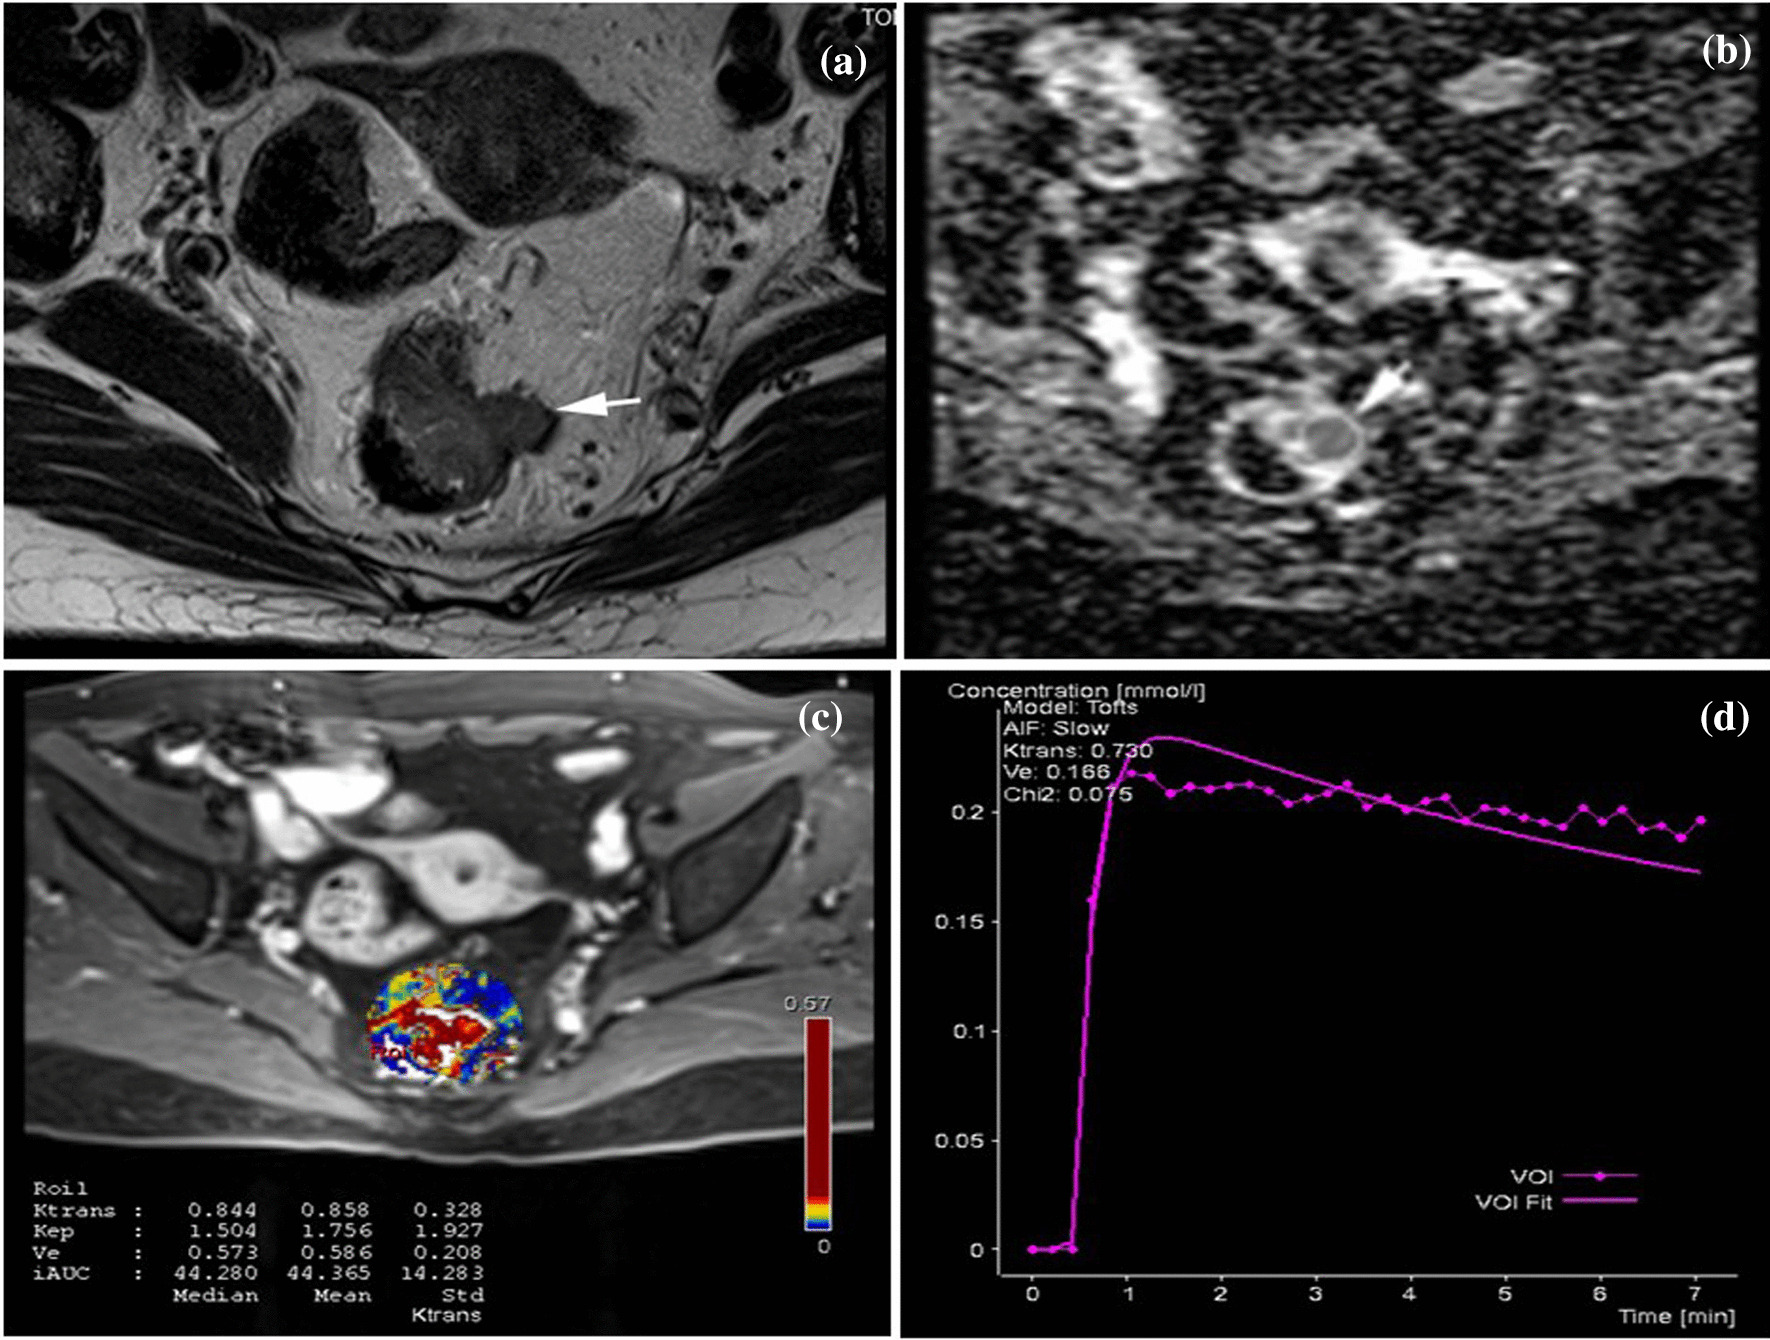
67-year-old woman with mrEMVI positive rectal cancer. a HR-T2WI showed rectal wall thickening and irregular nodular signal beyond the wall of rectum. ADC (b) and perfusion (c) image of the rectal cancer segment showing ROI placement and DCE-MRI quantitative parameters values. d Time-signal intensity curve was washout type
